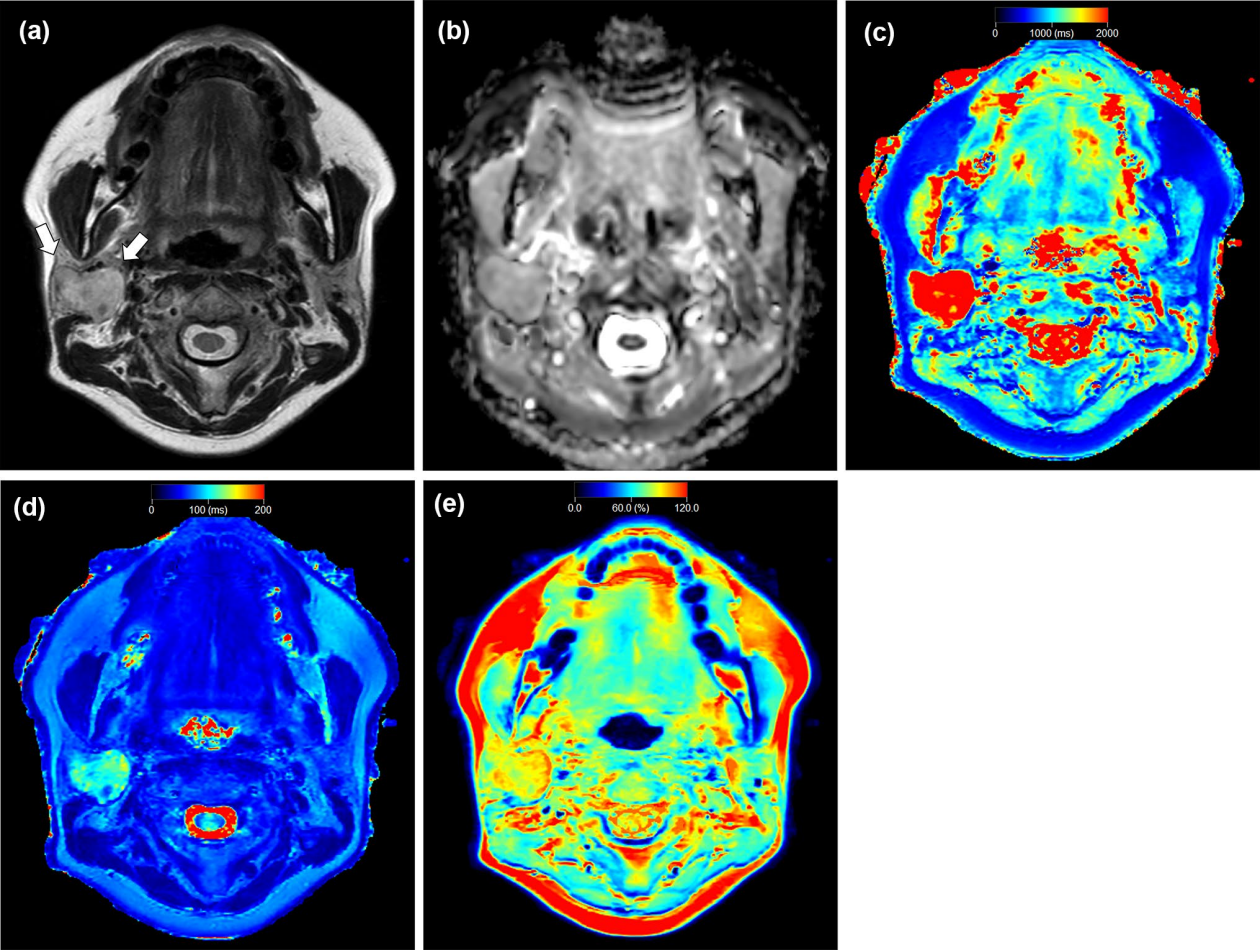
A 49-year-old female with pleomorphic adenoma. T2-weighted image (a) shows a lesion with higher signal intensity (arrow) relative to parenchyma in the adjacent right parotid gland. Apparent diffusion coefficient (ADC) (b), T1 (c), T2 (d), and PD (e) maps show the lesion, which has a mean ADC value of 1.49 × 10^−3^ mm^2^/s, mean T1 value of 2520 ms, mean T2 value of 103 ms, and mean PD value of 89%
